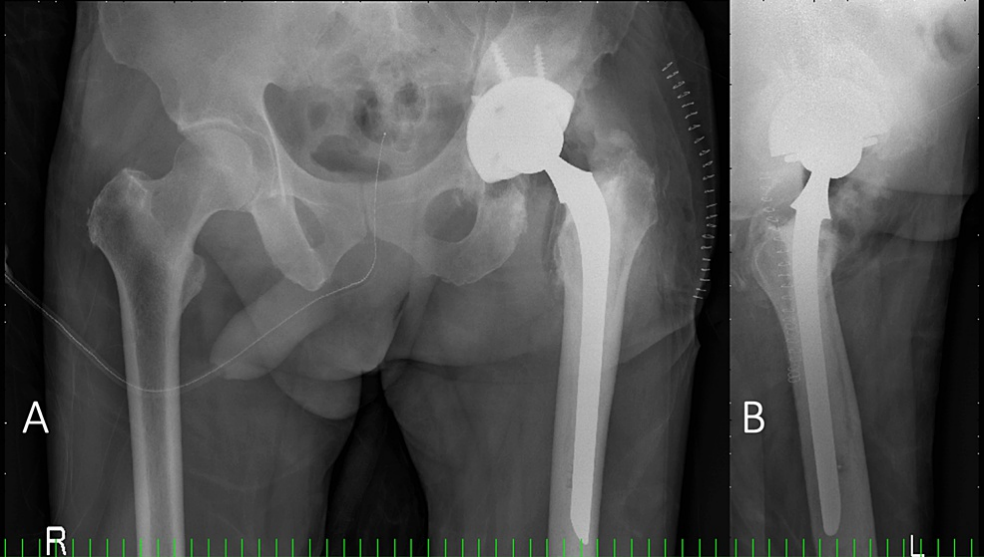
Plain radiograph after the second revision surgery A) Frontal radiograph; B) Lateral radiograph
